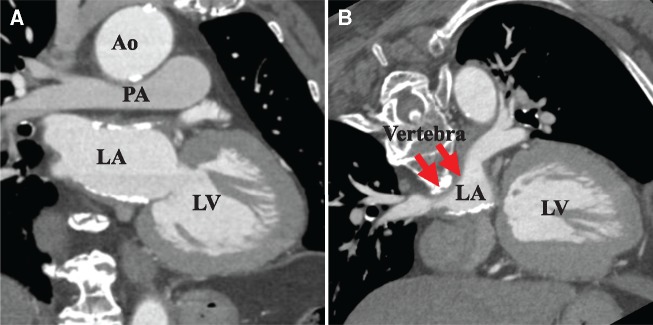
Multiplanar reconstruction images from multidetector row computed tomography are shown. The left atrium is sandwiched between dense calcification (*A*). Moreover, the posterior wall (red arrow) is compressed by the vertebral body (*B*). LA, left atrium; VB, vertebral body.
